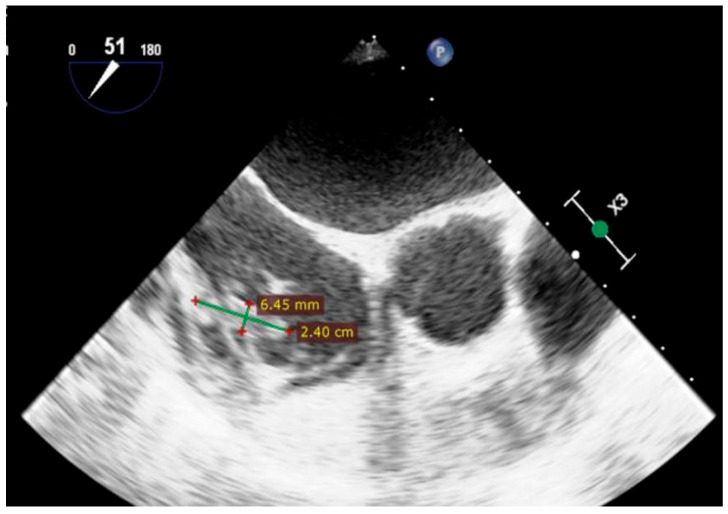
Two-dimensional transesophageal echocardiogram shows a large vegetation attached to one of the pacemaker leads, measuring 24 mm × 6.45 mm. The vegetation is measured and indicated with green crosses.
